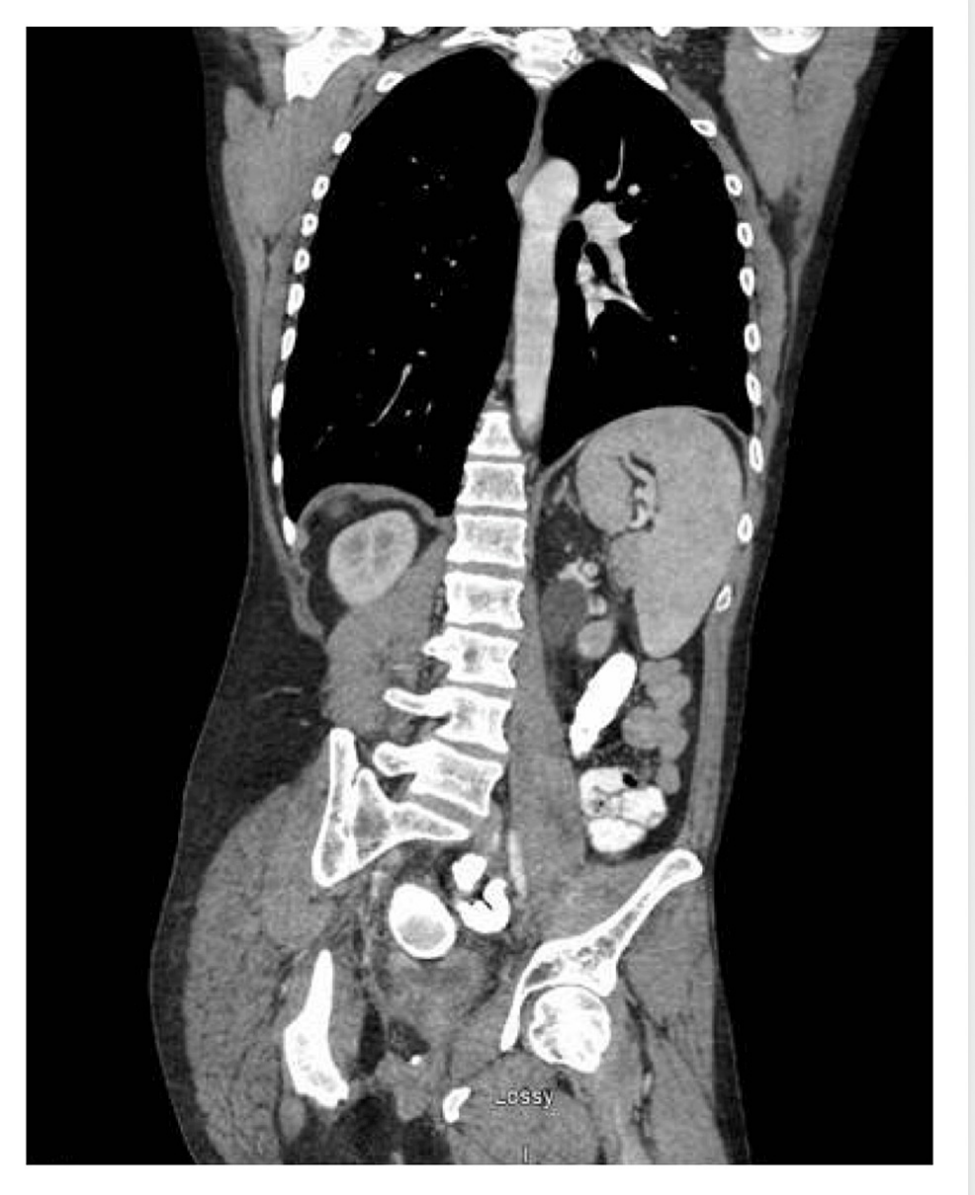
CT abdomen pelvis showing post-surgical changes and no evidence of recurrent disease Coronal view
